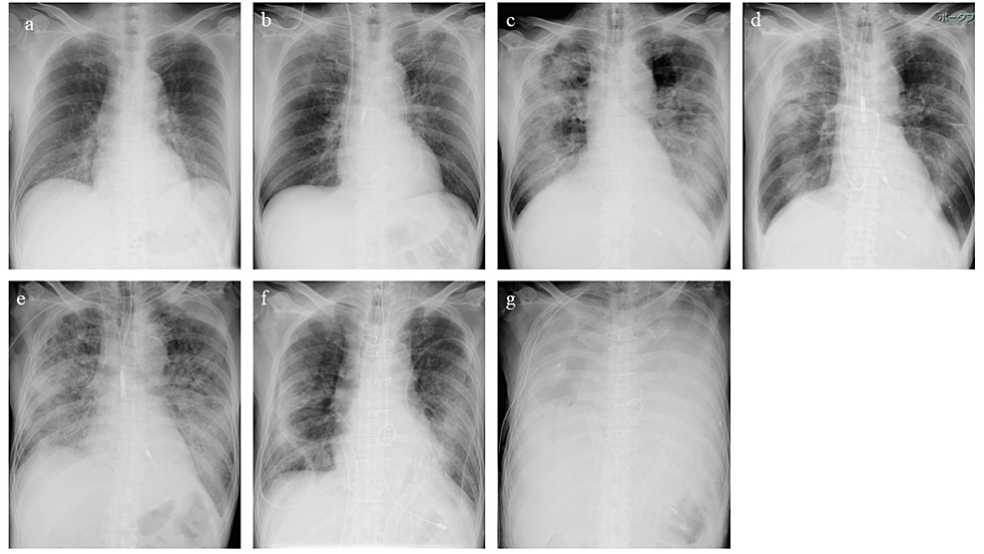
Changes in chest X-ray findings a; on admission, b; after Impella 2.5, c; before Impella 5.0, d; after Impella 5.0, e; before ECPELLA (adding veno-arterial extracorporeal membrane oxygenation (VA-ECMO) on Impella 5.0), f; after hybrid VA-ECMO, g; after extubation hybrid VA-ECMO
